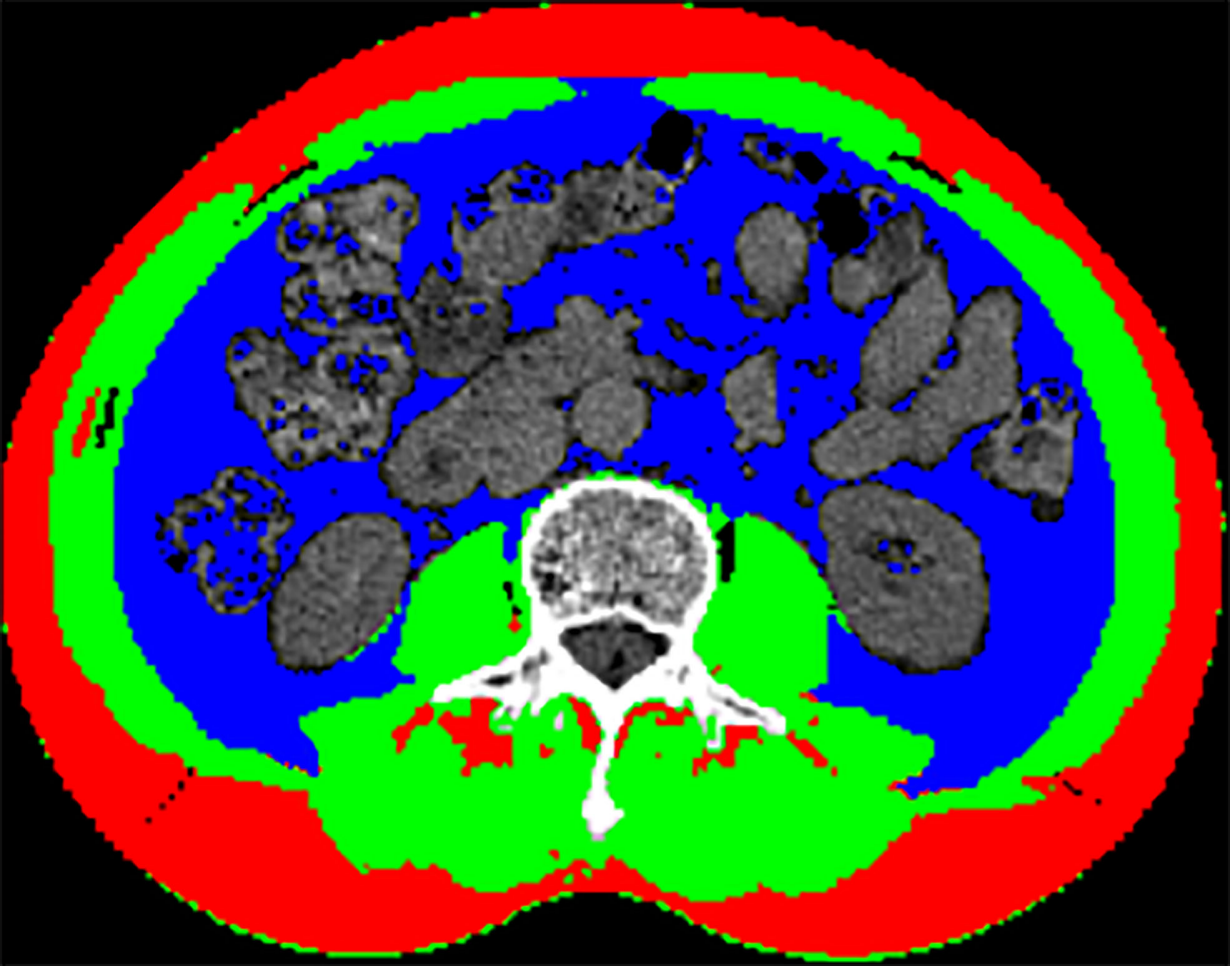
Representative CT image of the skeletal muscle area at L3 level: (Green) skeletal muscle; (Blue) visceral adipose tissue; (Red) subcutaneous adipose tissue.
